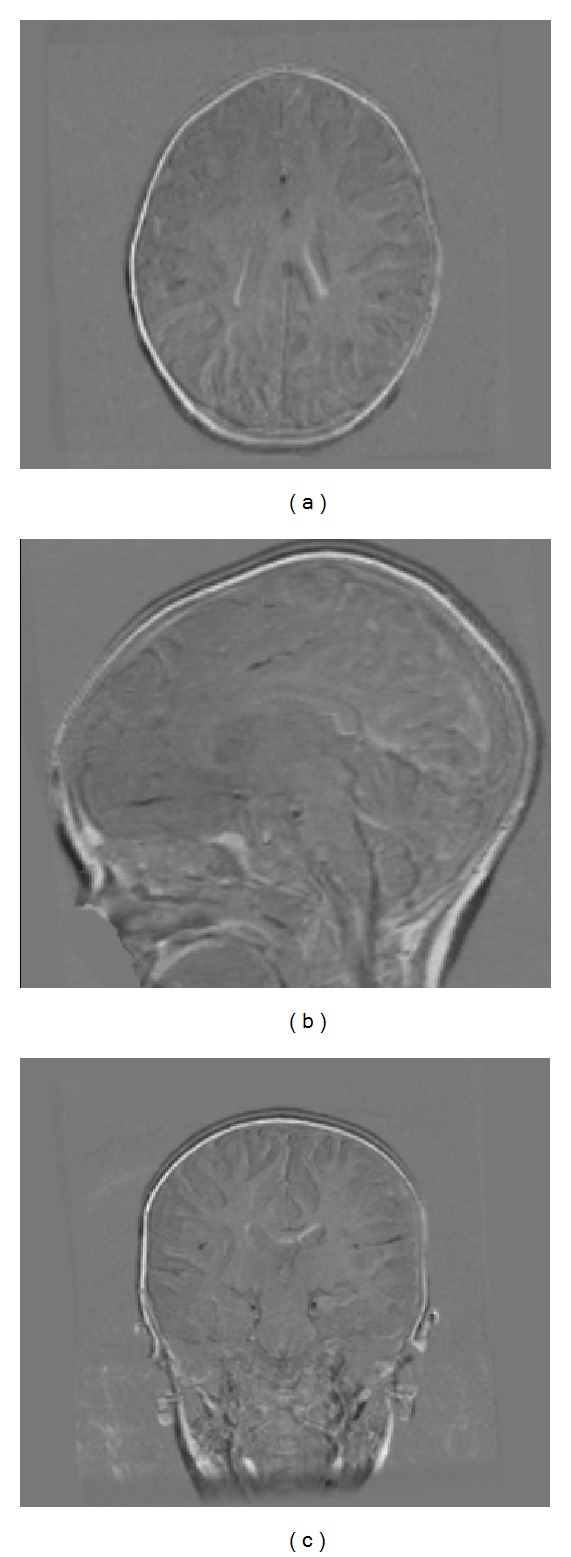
Image difference between the T1-weighted volumes for one of the subjects scanned at 2 and 4 years old. (a) Axial (b) sagittal, and (c) coronal views.
